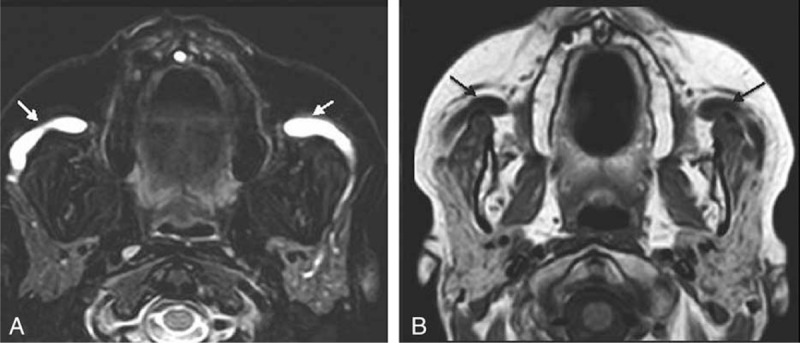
(A) MRI fat saturated T2-weighted axial plane. (B) MRI T1-weighted axial plane. The parotid glands have a conserved signal and size. There is no evidence of intaparotid solid or cystic lesions. There is significant dilation of the main drainage ducts (arrows). MRI = magnetic resonance imaging.
